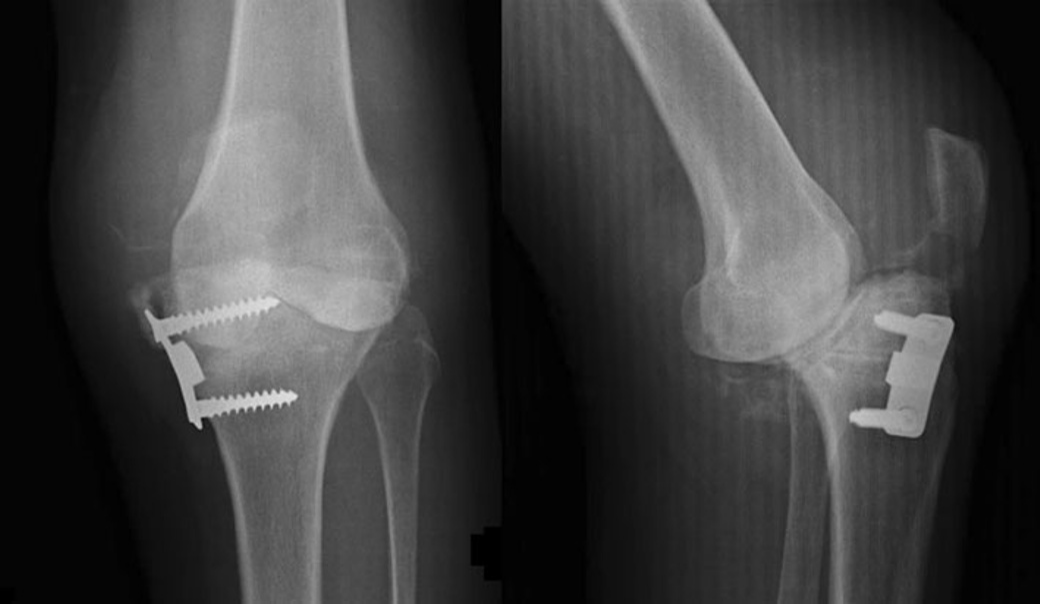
X-rays of the left knee at 8 months follow-up showing an anterior knee subluxation, osseous debris, and joint effusion
